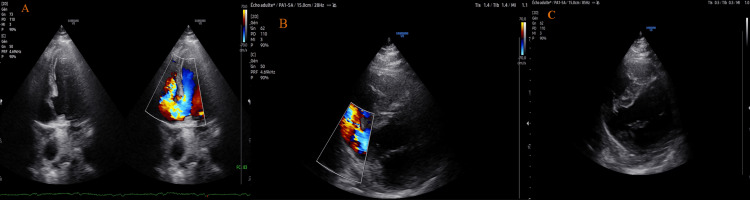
Transthoracic echocardiogram (A) Apical four-chamber view with color Doppler demonstrating ventricular septal rupture; (B) Parasternal short-axis view with color Doppler showing left-to-right shunt across the rupture; (C) Parasternal short-axis view revealing inferior wall rupture contained by the pericardium.
